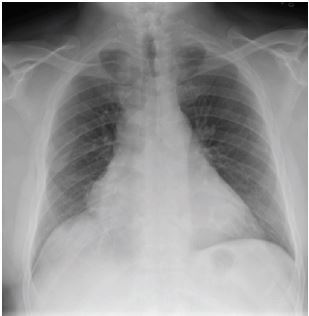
Chest radiograph of a 55-year-old male on hemodialysis who presented to the emergency department with fatigue.
